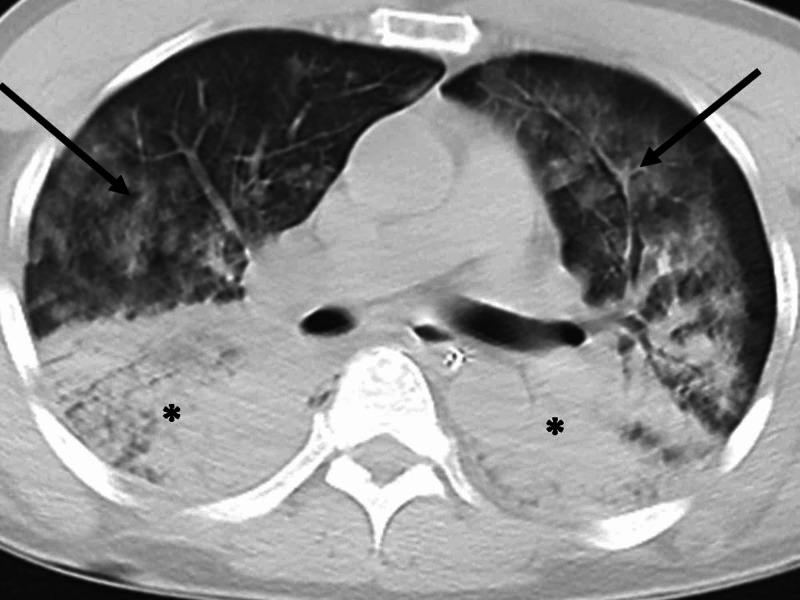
CT on arrival The image shows a bilateral ground-glass appearance in the ventral lung fields (arrow) and bilateral consolidation in the dorsal lung fields (asterisks) CT: computed tomography
